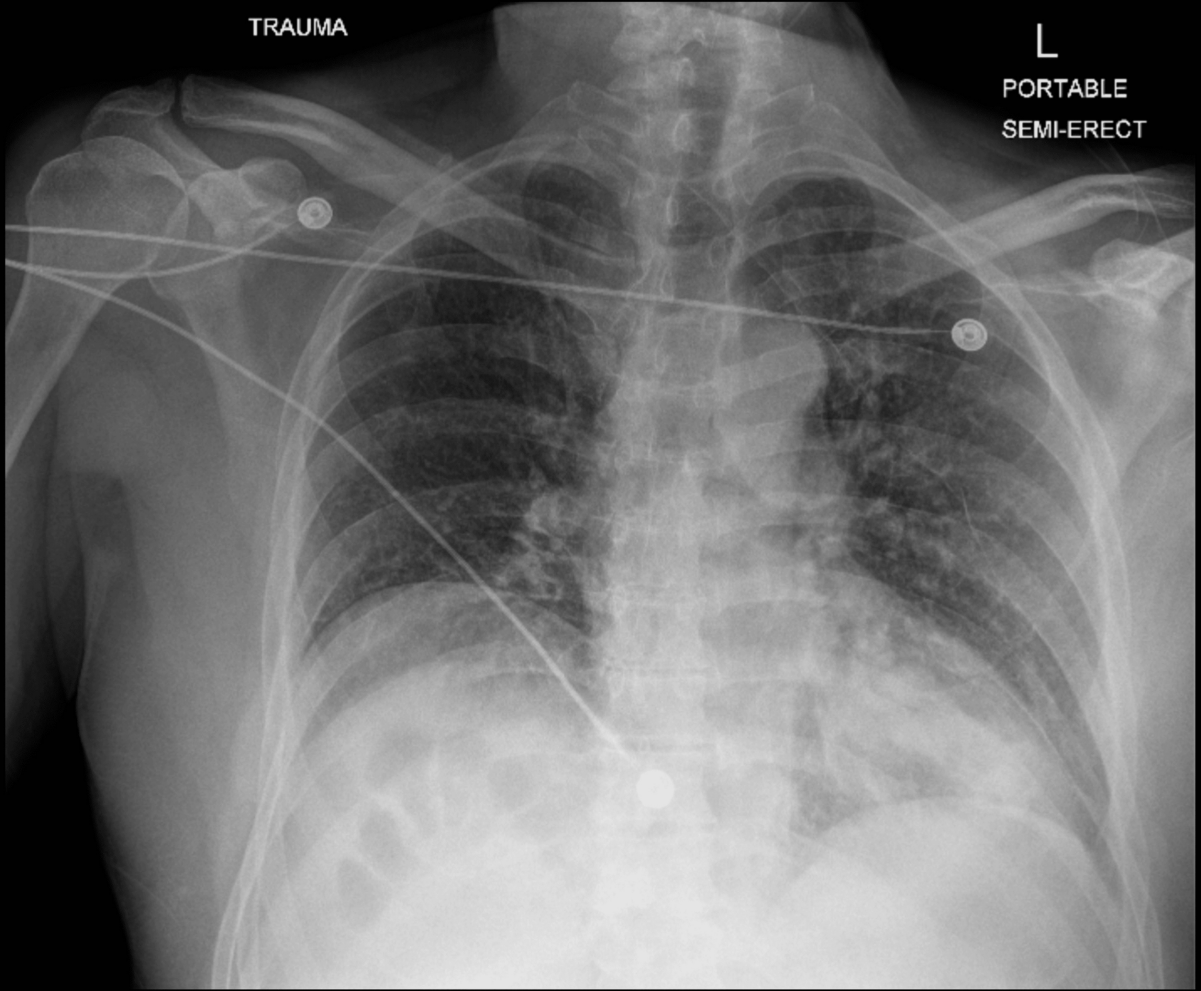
Frontal chest X-ray of a trauma patient showing no evidence of active cardiopulmonary disease.
